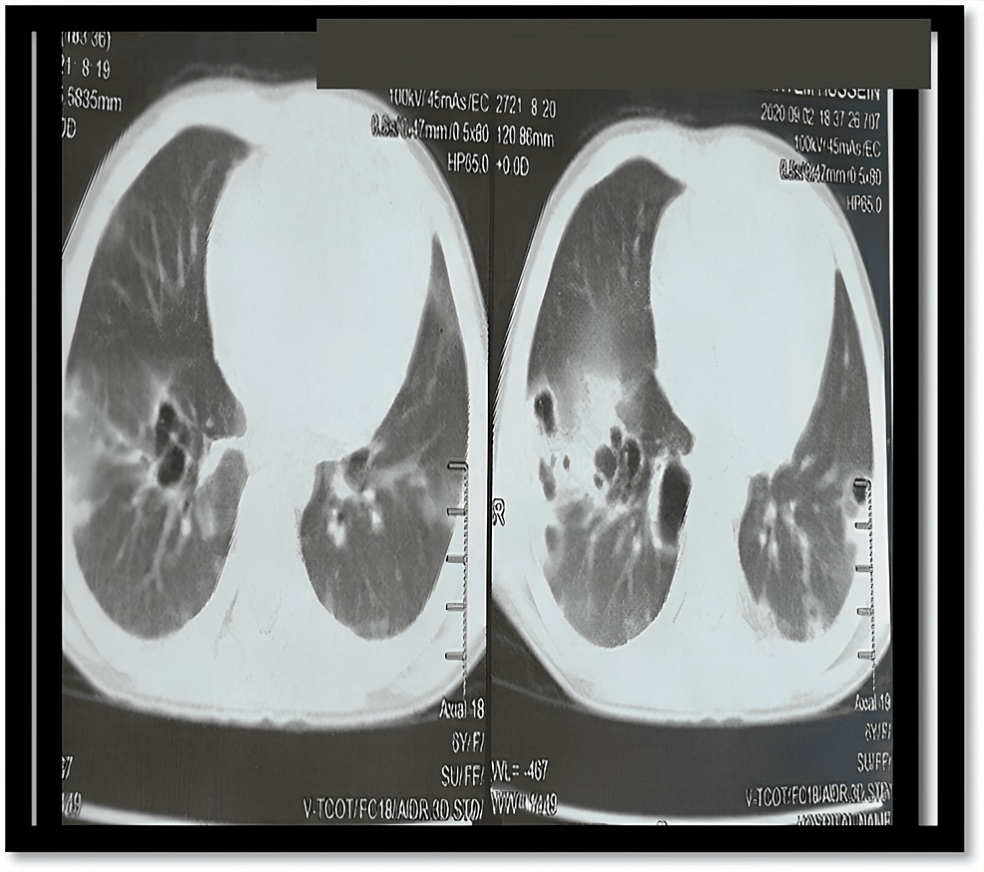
Axial computed tomography of the lungs of the patient on admission
